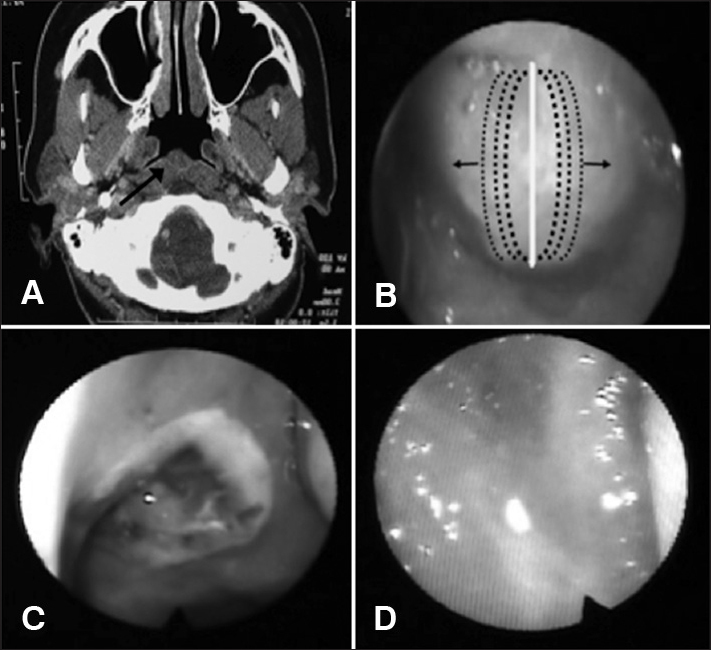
A: CT scan of the sinuses showing a lesion in the nasopharynx; B: Direction of the vaporization of the free edges of the cyst; C: Appearance of the surgical injury on the second day postoperatively; D: Three months postoperatively, normal looking mucosa without signs of recurrence.
